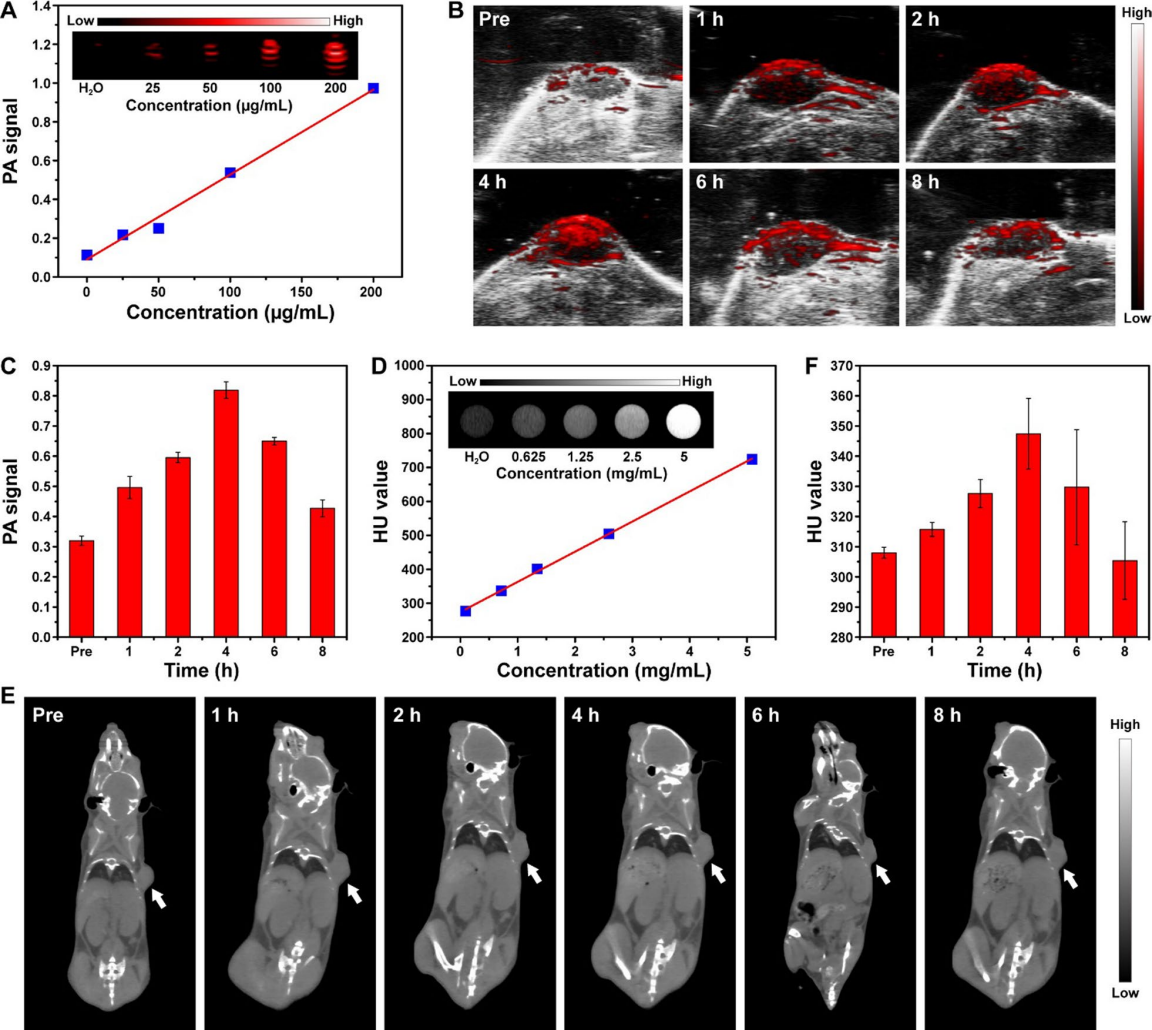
A In vitro PA images and corresponding signal intensities of Au@Cu_2-x_Se aqueous dispersions with different concentrations. B In vivo PA images of tumor and C corresponding signal intensities obtained at different time points after intravenous injection of Au@Cu_2-x_Se NPs (2 mg mL^−1^ in saline, 200 µL). D In vitro CT images and corresponding HU values of Au@Cu_2-x_Se aqueous dispersions with different concentrations. E In vivo CT images of tumor (indicated by white arrow) and F corresponding HU values obtained at different time points after intravenous injection of Au@Cu_2-x_Se NPs (2 mg mL^−1^ in saline, 200 µL). Data are presented as mean ± s.d. (n = 3)
